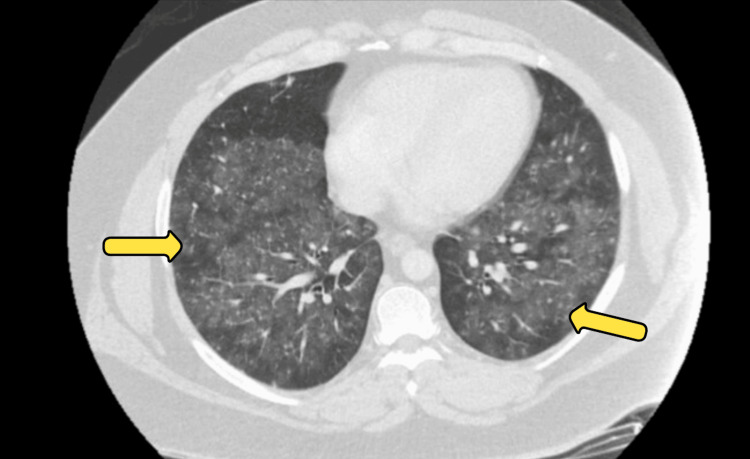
Axial CT chest demonstrating multifocal bilateral ground-glass opacities (arrows).
